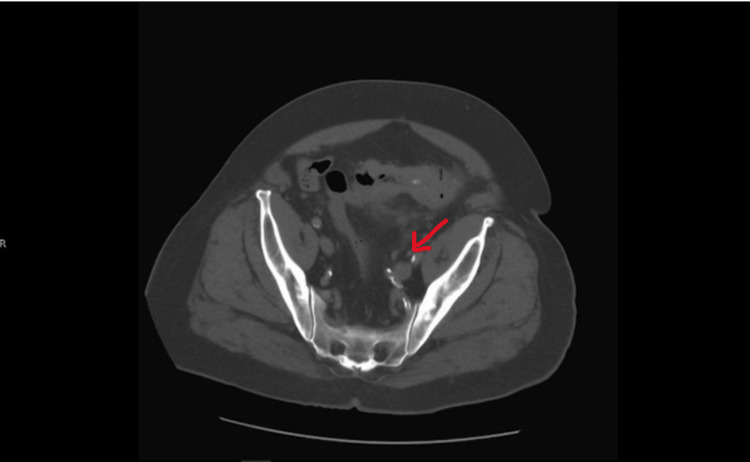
The ingested wishbone visualized radiographically in the transverse plane with intravenous contrast at the level of the sigmoid colon, demonstrating a proximal thickened bowel segment.
